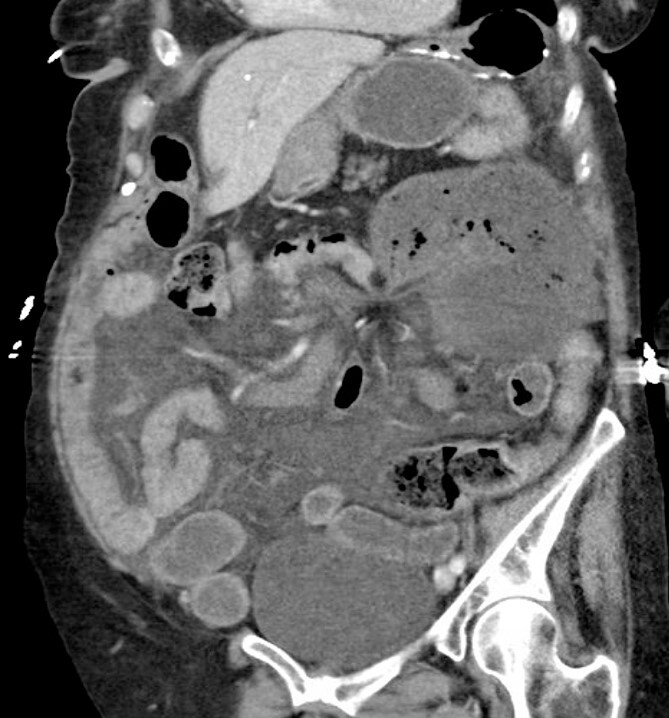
Computed tomography showing cecal volvulus secondary to internal hernia.
